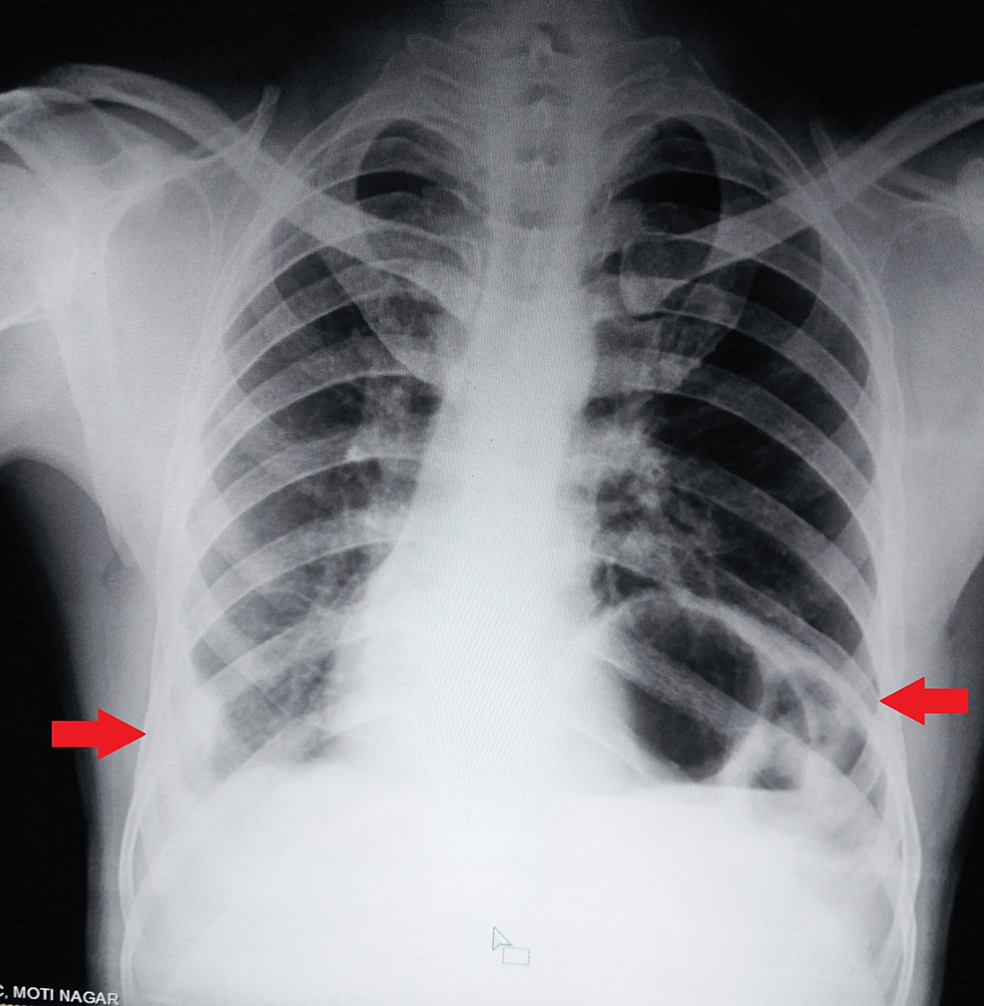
A follow-up chest radiograph showing that there was no progression of disease. Arrows indicate a raised dome of the left diaphragm and blunting of the costophrenic angle on the right or right pleural effusion.
